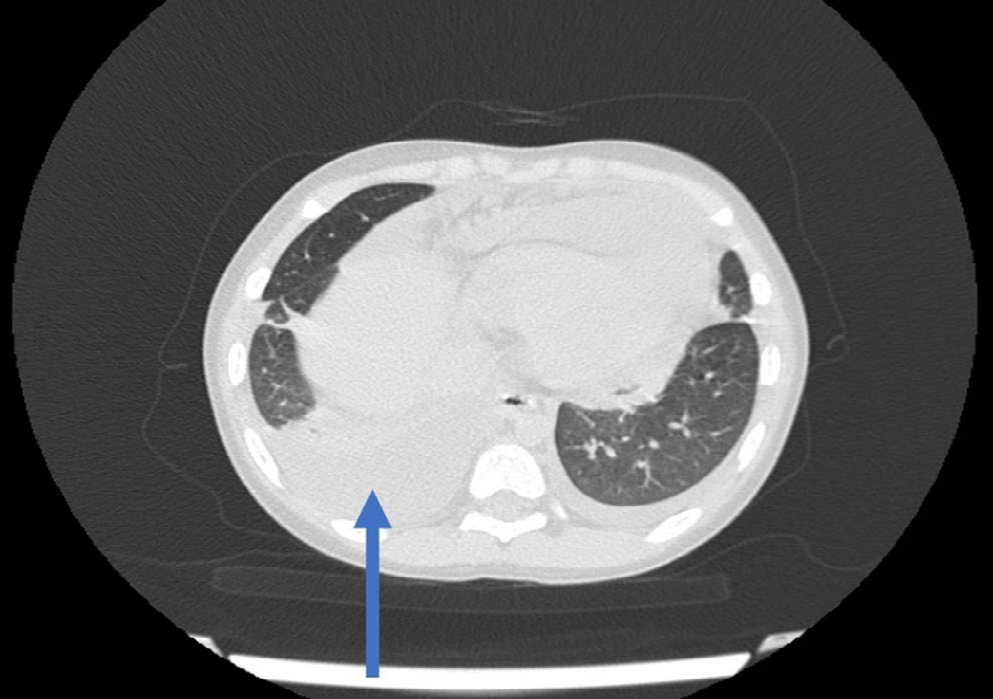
Thorax computed tomography (CT) scan revealed a mediastinal mass accompanied by pleural and pericardial effusion (arrows)
